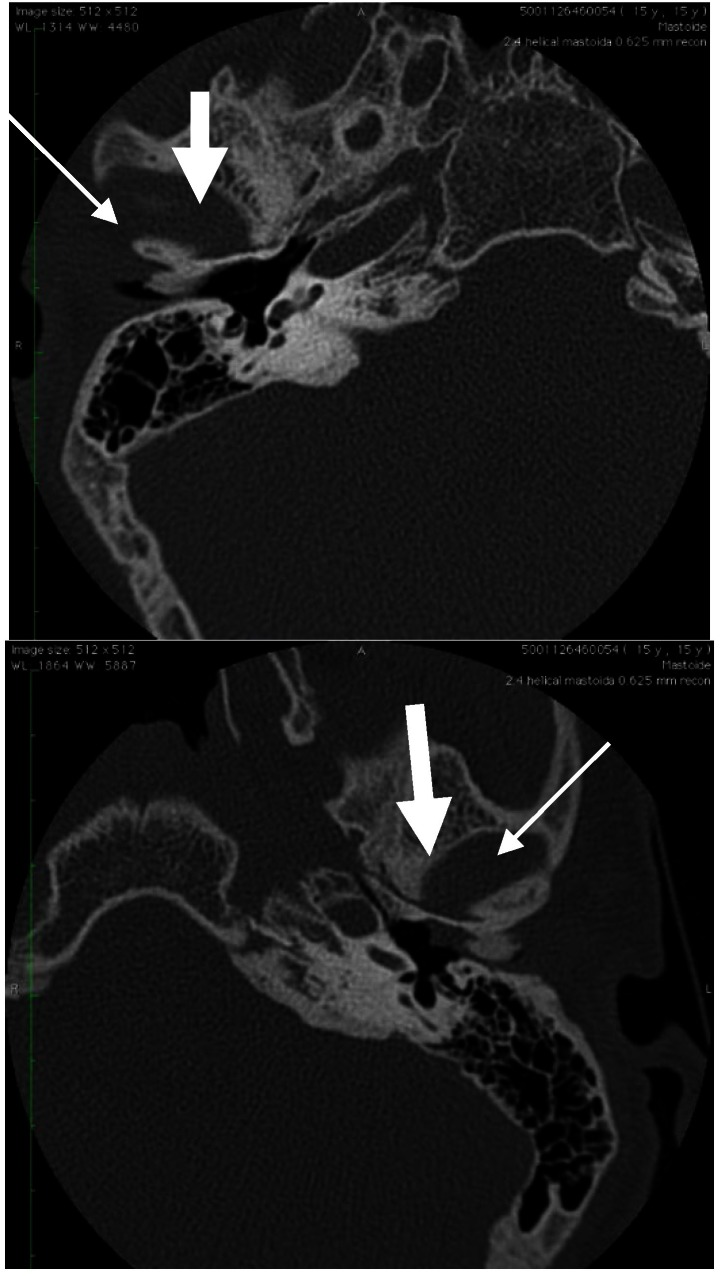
CT-scan revealing external ear osteomas on both sides, with insertion of the ear canal (thin arrows) on the anterior wall; the space outside TM on the right side is clear; the same space on the left ear is full (thick arrows)
